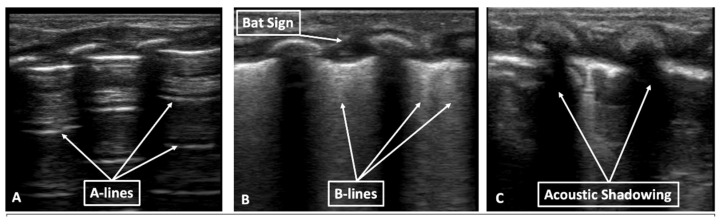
Lung ultrasound in B-mode. (A) A-lines, reverberation artifact from pleural line (B) B-lines, reverberation artifact from interstitial edema; (C) acoustic shadowing from the ribs.
